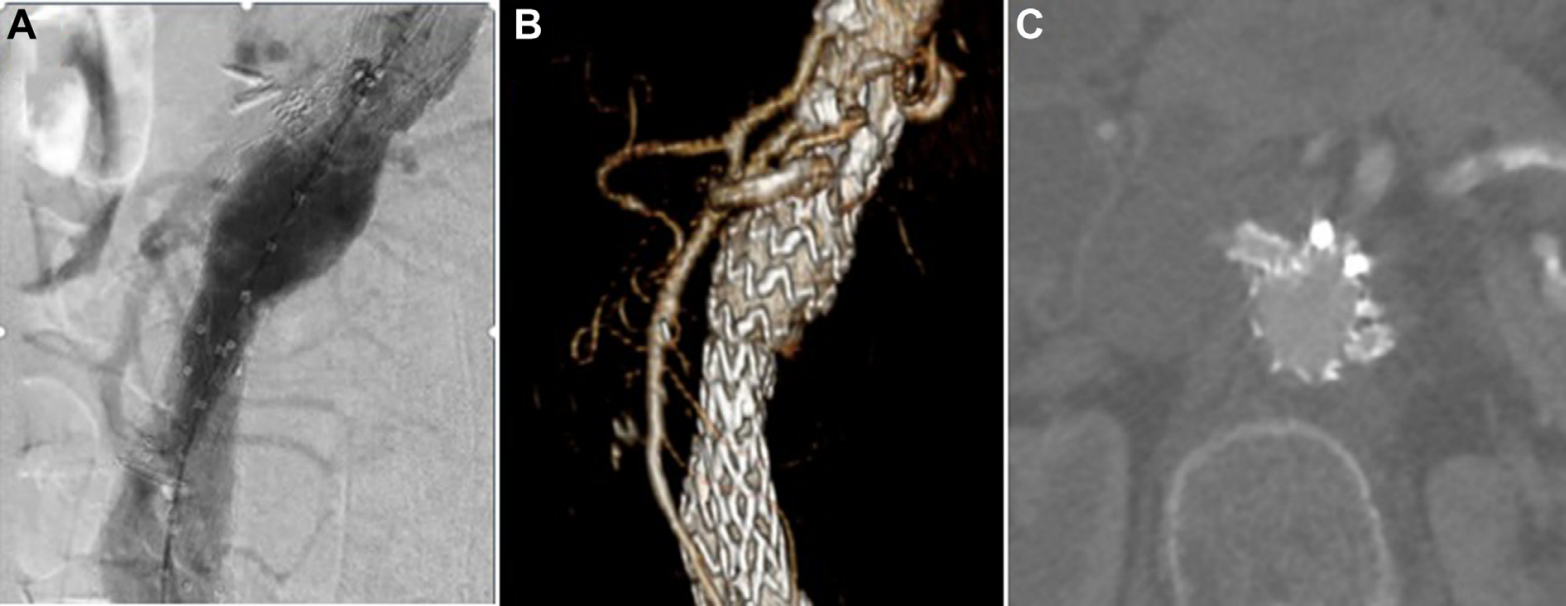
A, No endoleak on completion angiogram. B and C, Follow-up computed tomography angiogram shows renal arteries widely patent.
